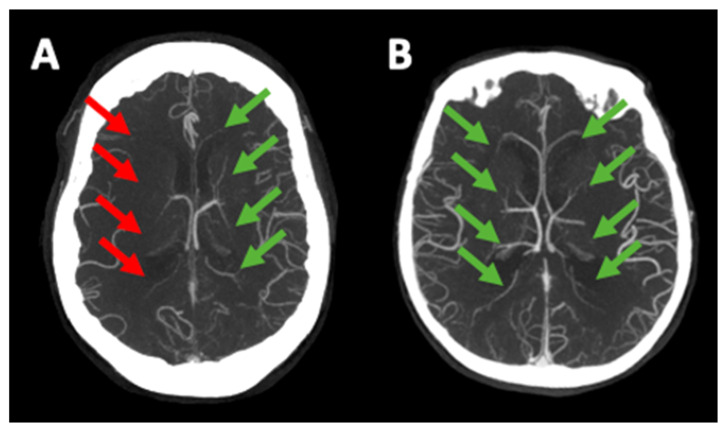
Multiphase CT-angiography comparison between unfavorable (A) and favorable (B) deep medullary vein profiles. Red arrows indicate a lack of venous opacification, while green arrows show full vein opacification.
